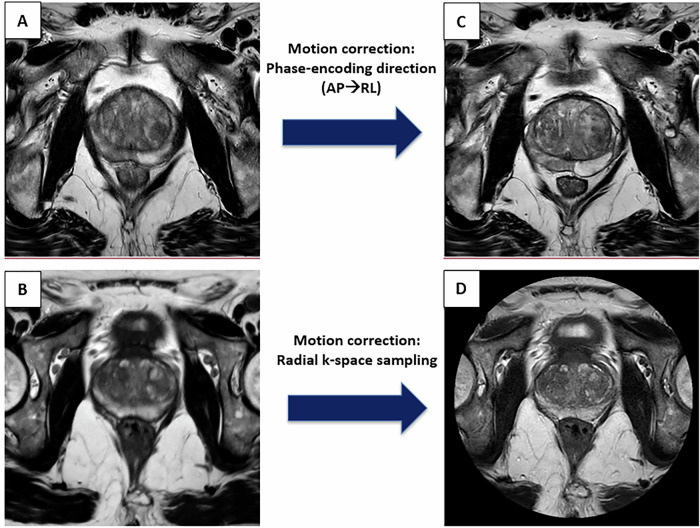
Effect of motion correction techniques on axial T2-weighted imaging. A, B Motion-related ghosting artefacts are seen as blurring and loss of sharp prostate margins. C Switching the phase-encoding direction from anterior–posterior (AP) to right–left (RL) reduces rectal motion artefacts, improving lesion conspicuity. D Radial k-space sampling (MultiVane XD) further mitigates ghosting by oversampling the k-space centre and redistributing motion effects, resulting in superior image quality. This example highlights how advanced acquisition strategies can substantially reduce motion-related artefacts in prostate MRI
